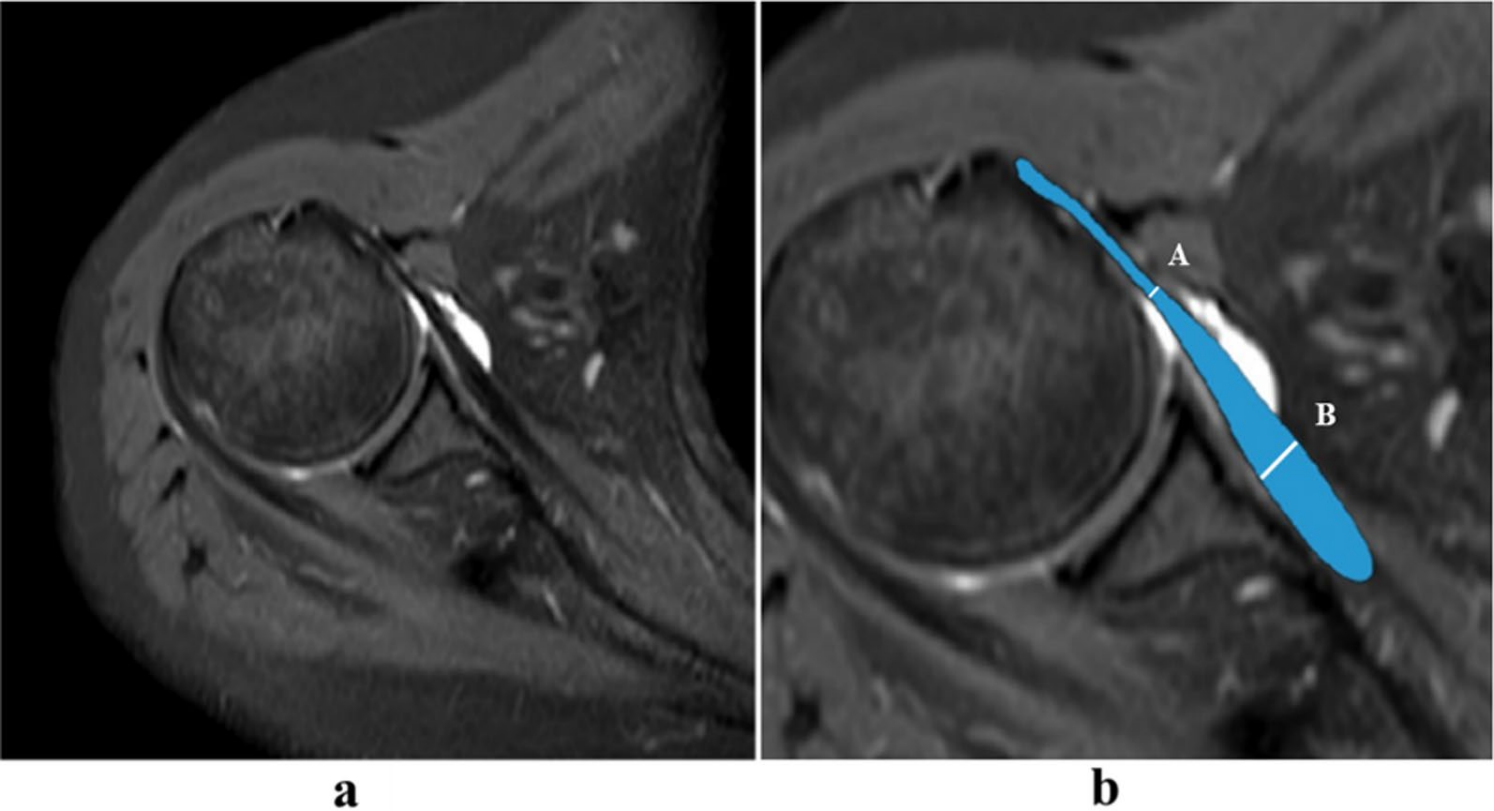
Evaluation of thinning of SSC tendon on axial IM-WI/fs MRI. Manually measuring the diameter of the SSC tendon at its thinnest proximal point (a) and its thickest distal point (b), and the ratio of a and b is < 1/3 as the reference value of thinning of the SSC tendon
